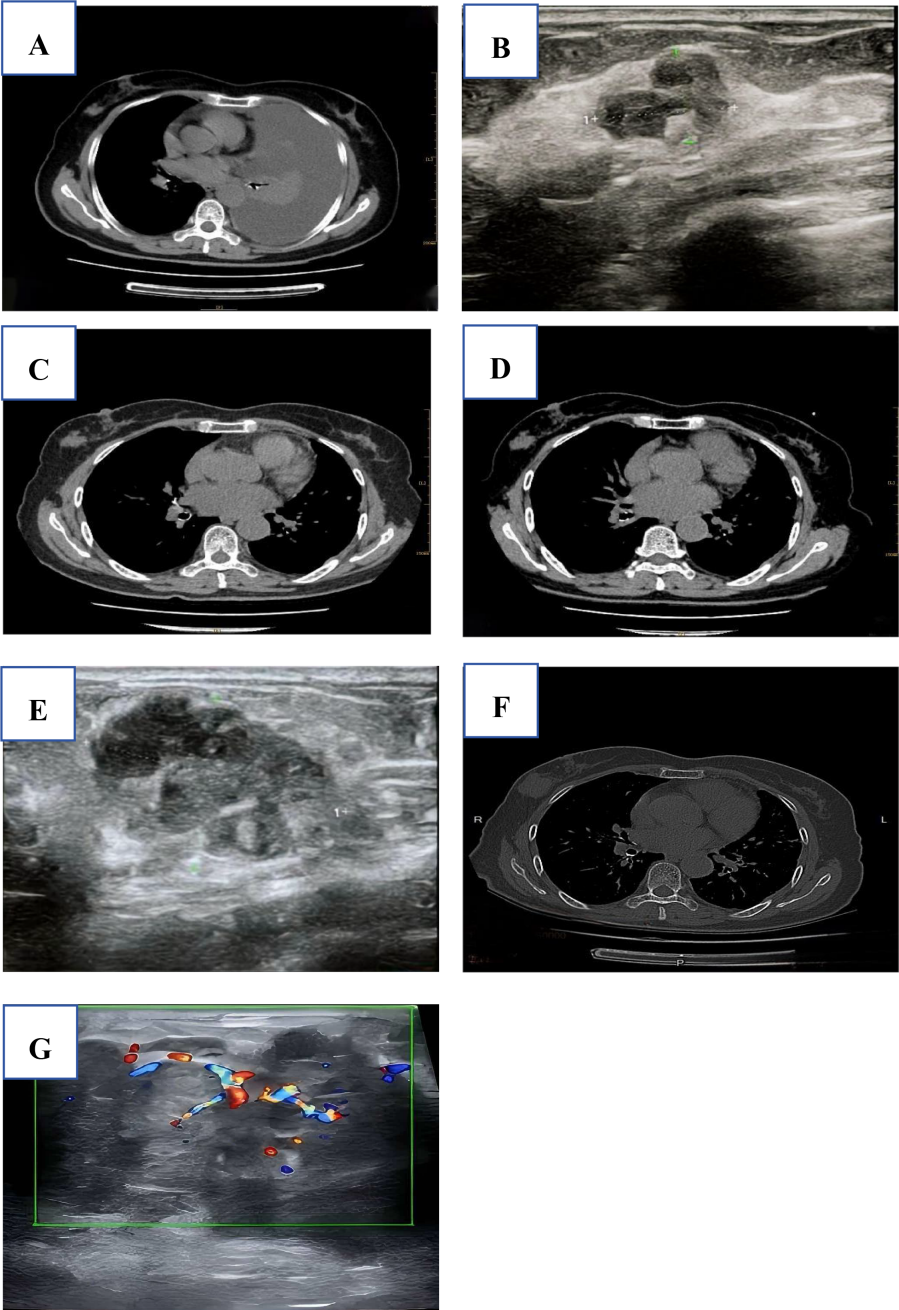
(A) 2019-07-02, CT scan: A well - defined nodule measuring 13.9 mm × 10.1 mm was identified in the upper outer quadrant of the right breast. (B) 2019-10-15, Breast Ultrasound: A hypoechoic nodule measuring 14.1 mm × 10.2 mm was detected in the right breast, classified as BI - RADS category 4a. (C) 2020-10-09, CT scan: A 21 mm ×18 mm nodular opacity was observed in the upper outer quadrant of the right breast; its etiology remains indeterminate and requires further characterization. (D) 2021-03-31, CT scan: A 23.3 mm × 18.6 mm nodular opacity was present in the upper outer quadrant of the right breast, exhibiting irregular morphology, relatively well - defined margins, and mild post-contrast enhancement; no significant interval change is observed compared to the prior study. (E) 2022-02-08, Breast Ultrasound: A 27.2 mm × 18.7 mm slightly hypoechoic mass was observed in the right breast, classified as BI - RADS category 4a. (F) 2022-11-08, CT scan: A 30.3 mm × 29.0 mm nodular opacity was present in the upper outer quadrant of the right breast. (G) 2024-04-02, Breast Ultrasound: A 59 mm × 36 mm × 42 mm slightly hypoechoic mass was observed in the breast, exhibiting irregular morphology, poorly defined margins, and heterogeneous internal echotexture.
